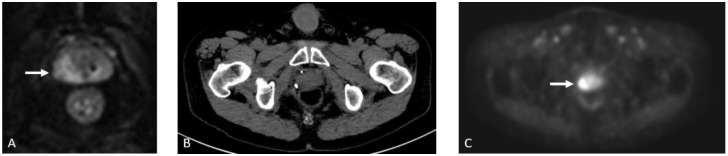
Eighty-four-year-old male with elevated PSA levels. PET image shows increased uptake within the right lateral peripheral zone concerning for recurrence. Corresponding CT shows no abnormality in the area of pathology. Magnetic resonance imaging (MRI) Diffusion-Weighted-Imaging (DWI), (A) sequence through the prostate shows increased DWI signal in the right mid-gland (arrow), PiRads 5. NECT (B) shows no discernable abnormality in the area of interest; however, corresponding Axumin PET (C) shows intense Axumin uptake (arrow).
